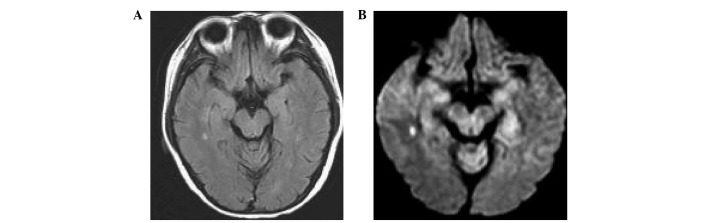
Case 2: (A) Axial T2 fluid-attenuated inversion recovery (FLAIR) exhibits spot-like hyperintensity, and (B) axial diffusion-weighted imaging (DWI) exhibits hyperintense foci at the right temporal lobe.
